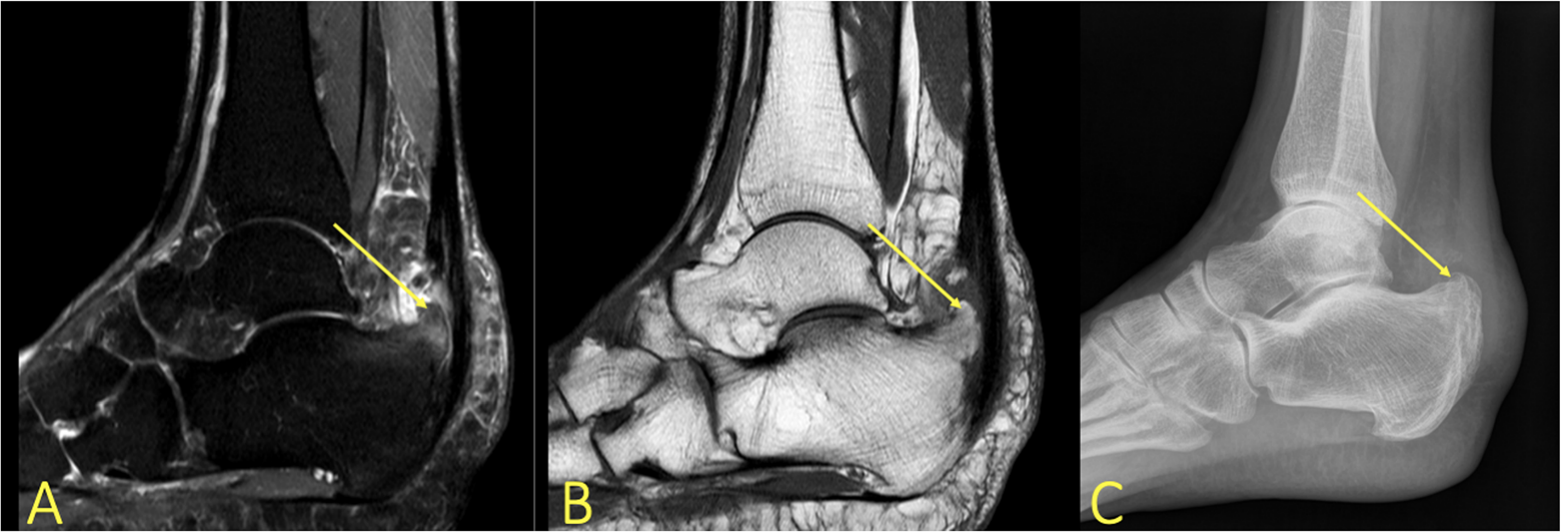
A 62-year-old female with recurring symptoms after previous surgery for a Haglund deformity. MRI and radiography showed a Haglund deformity (arrow) with bone marrow edema again visible on MRI
